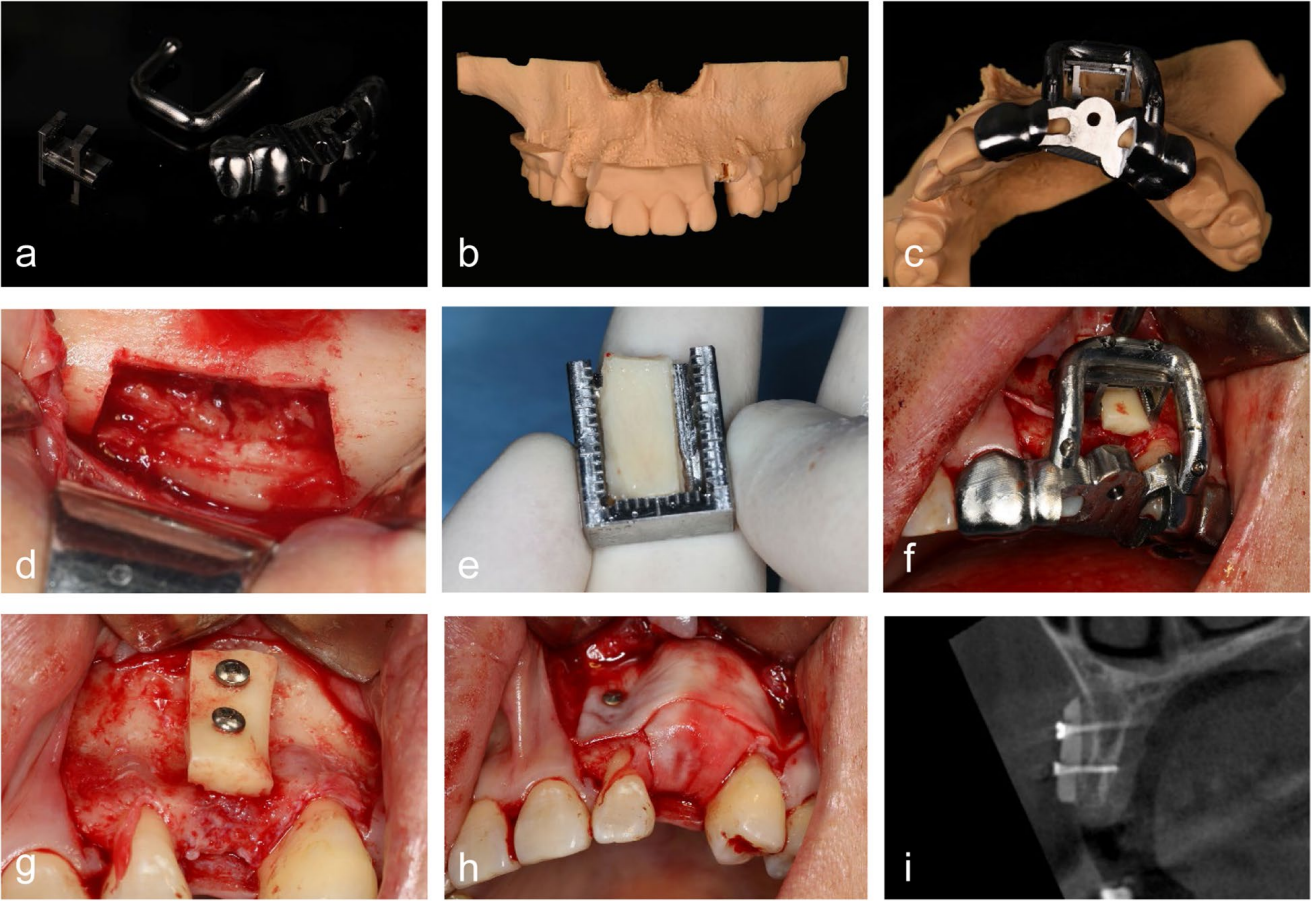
Onlay bone graft surgery. a Three parts of the graft template milled with titanium; b printed diagnostic cast; c template trying in diagnostic cast; d bone block extraction; e trimming of harvested bone block placed in trim template; f graft template positioned to complete drilling process; g bone block fixed with 2 screws; h the recipient area covered with resorbable collagen membrane; i postoperative CBCT imaging
